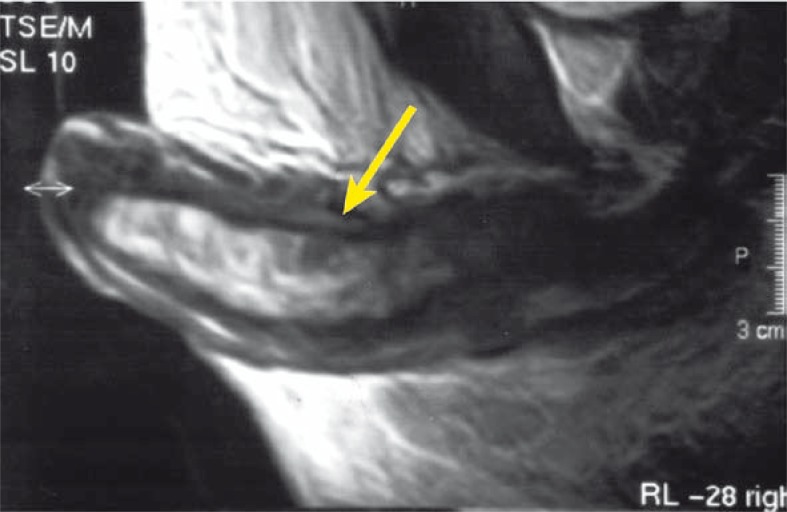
Sagittal T2-weighted MR image of the penis, demonstrating multiple metastatic nodules (arrow).
